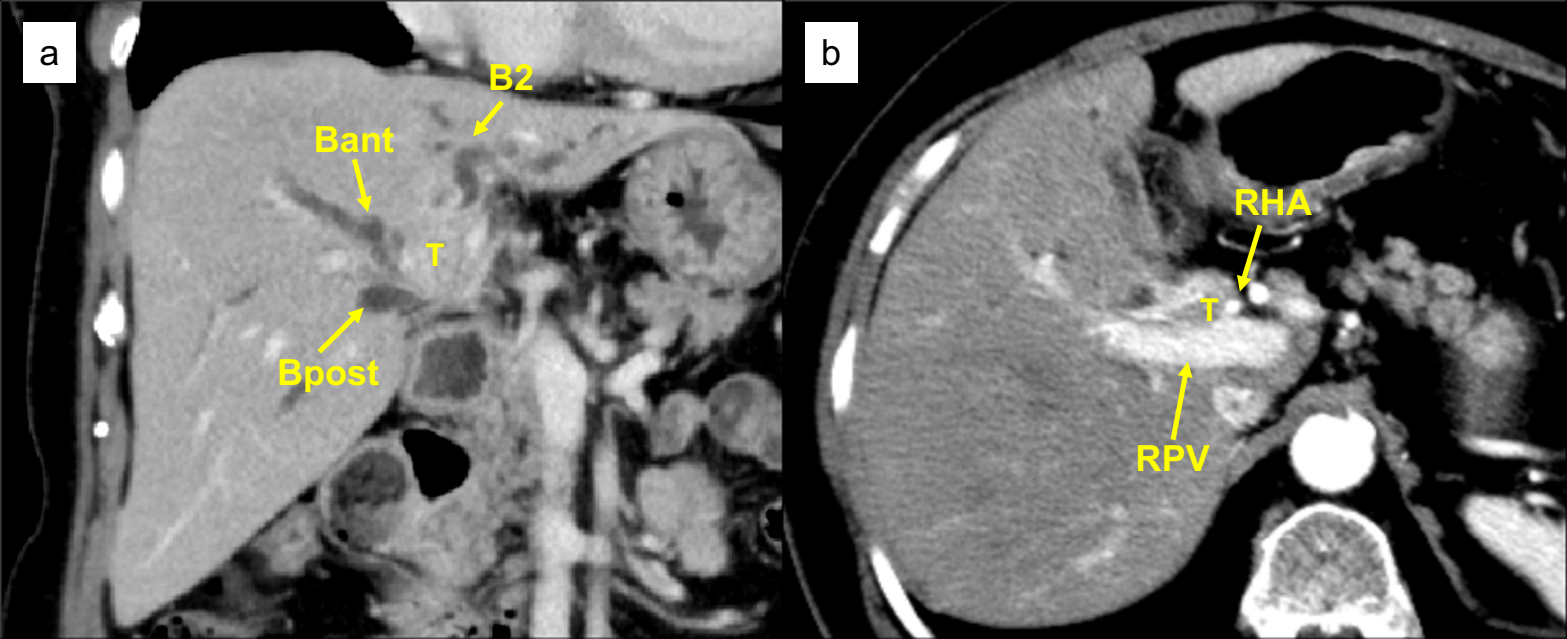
Preoperative contrast-enhanced computed tomography findings. The tumor (T) was mainly located in the left hepatic duct (a) and involved the right hepatic artery and the portal vein (b). *T* tumor, *B2* segment 2 branch of the bile duct, *Bant* anterior branch of the bile duct, *Bpost* posterior branch of the bile duct, *RHA* right hepatic artery, *RPV* right portal vein
